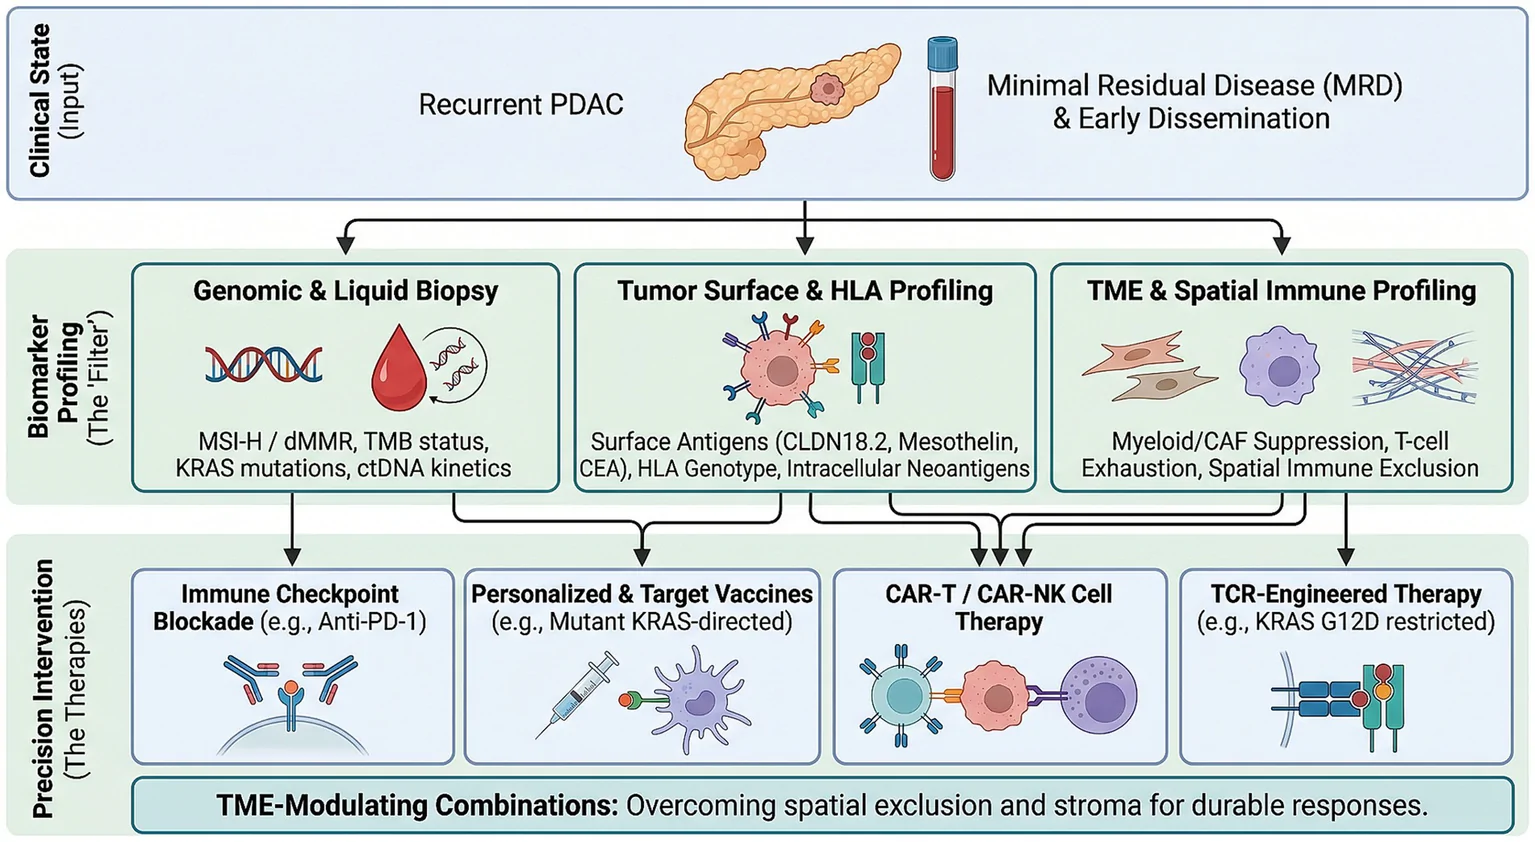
Biomarker-driven decision framework for immunotherapy and adoptive cell therapy in recurrent pancreatic ductal adenocarcinoma. The clinical sequence is: (1) Classify the recurrence state as molecular residual disease (MRD), limited radiographic relapse, disseminated recurrence, or refractory disease; (2) obtain a minimum biomarker panel using tissue and/or liquid biopsy; (3) match the patient to an evidence-appropriate intervention; (4) assess feasibility, including performance status, organ function, tissue availability, manufacturing time, access, and need for bridging therapy; and (5) monitor response and immune escape longitudinally.
